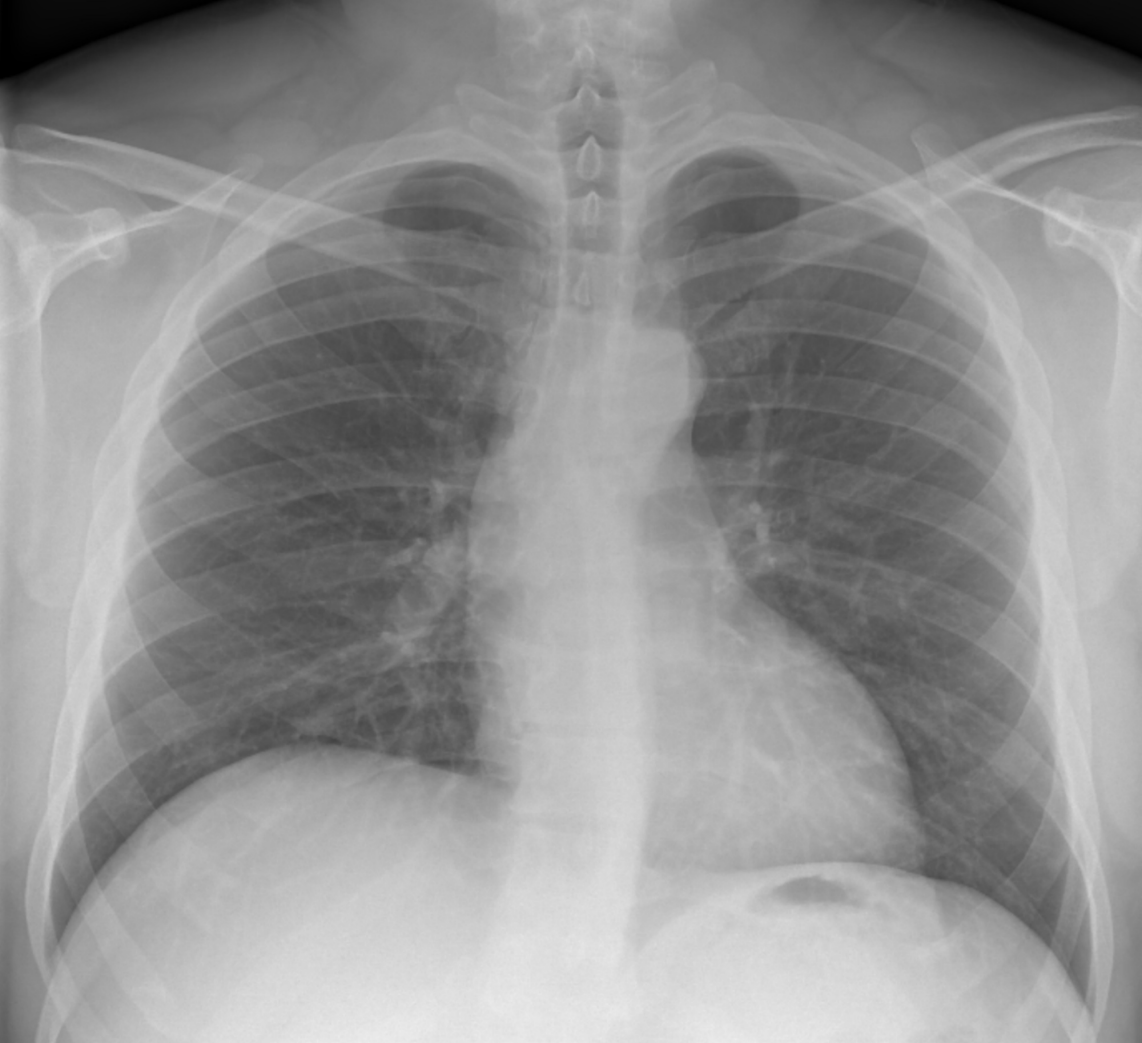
Chest X-Ray (PA) Unremarkable Chest X-Ray. Regular heart silhouette size and clear lung fields without pleura effusion, pulmonary edema, or vasculature congestion.
